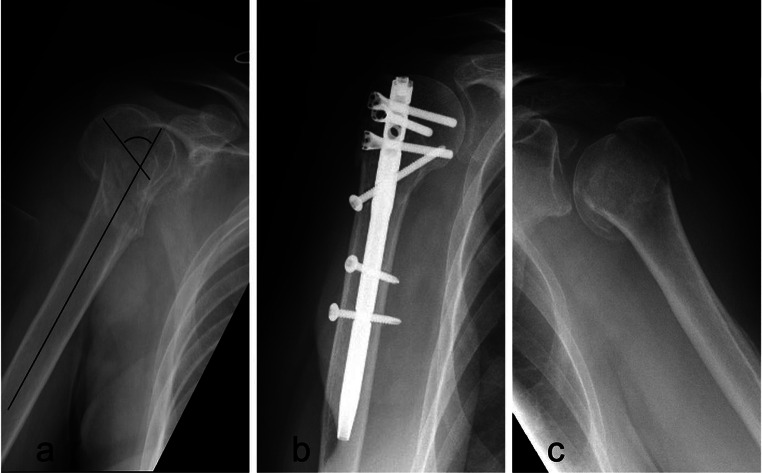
provides radiographs of each treatment modality. (a) A 69-year-old female patient presented with a 3-part fracture of her dominant right shoulder with significant valgus displacement of the head, displacement of the greater tuberosity (Neer type IV) and comminution of the calcar. (b) She underwent treatment with intramedullary nailing (IMN) using MultiLoc (DePuy Synthes). (c) A 77-year-old female patient from a nursing home presented with a varus-displaced impacted 3-part fracture (Neer type IV) and received non-operative treatment
